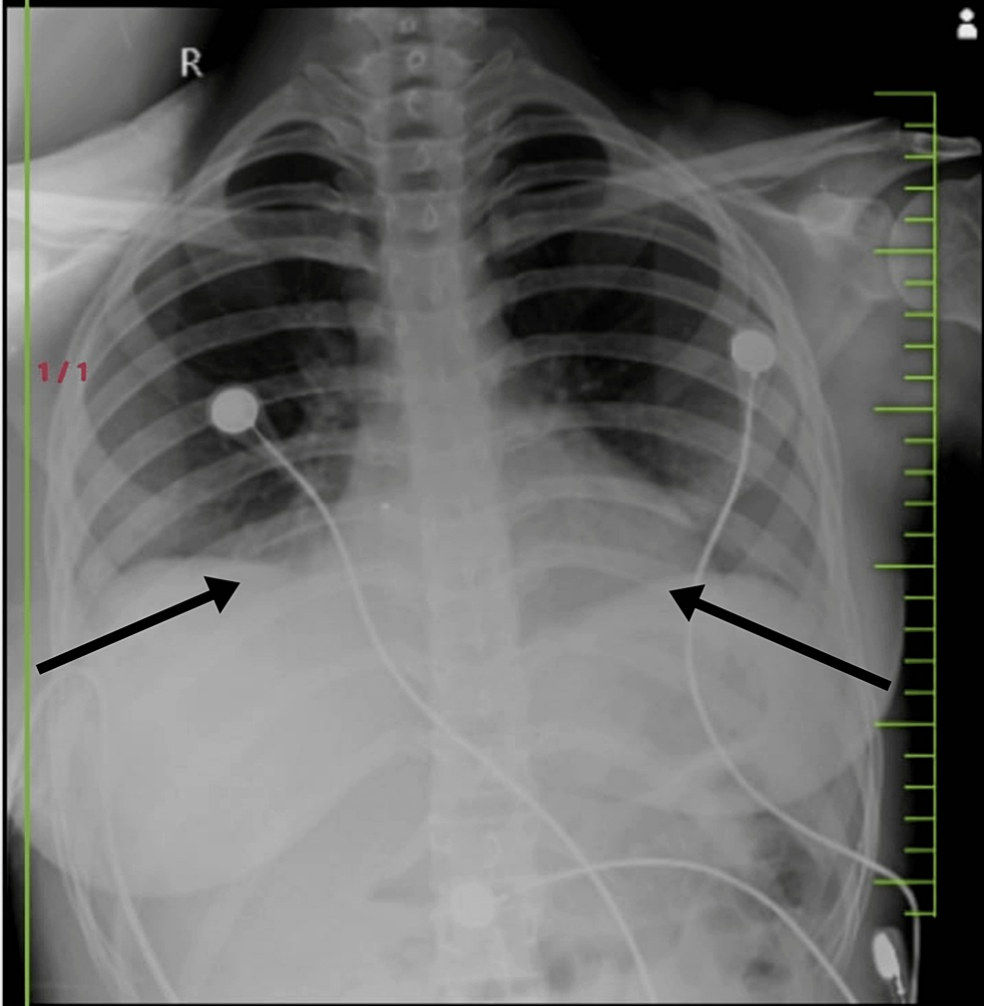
Chest X-ray on day 2 of admission. We can see that the costophrenic angles on bilateral lungs are obliterated. The chest X-ray indicates mild bilateral pleural effusion. The X-ray was advised after the patient started complaining of dyspnoea owing to her growing ovarian teratoma.
